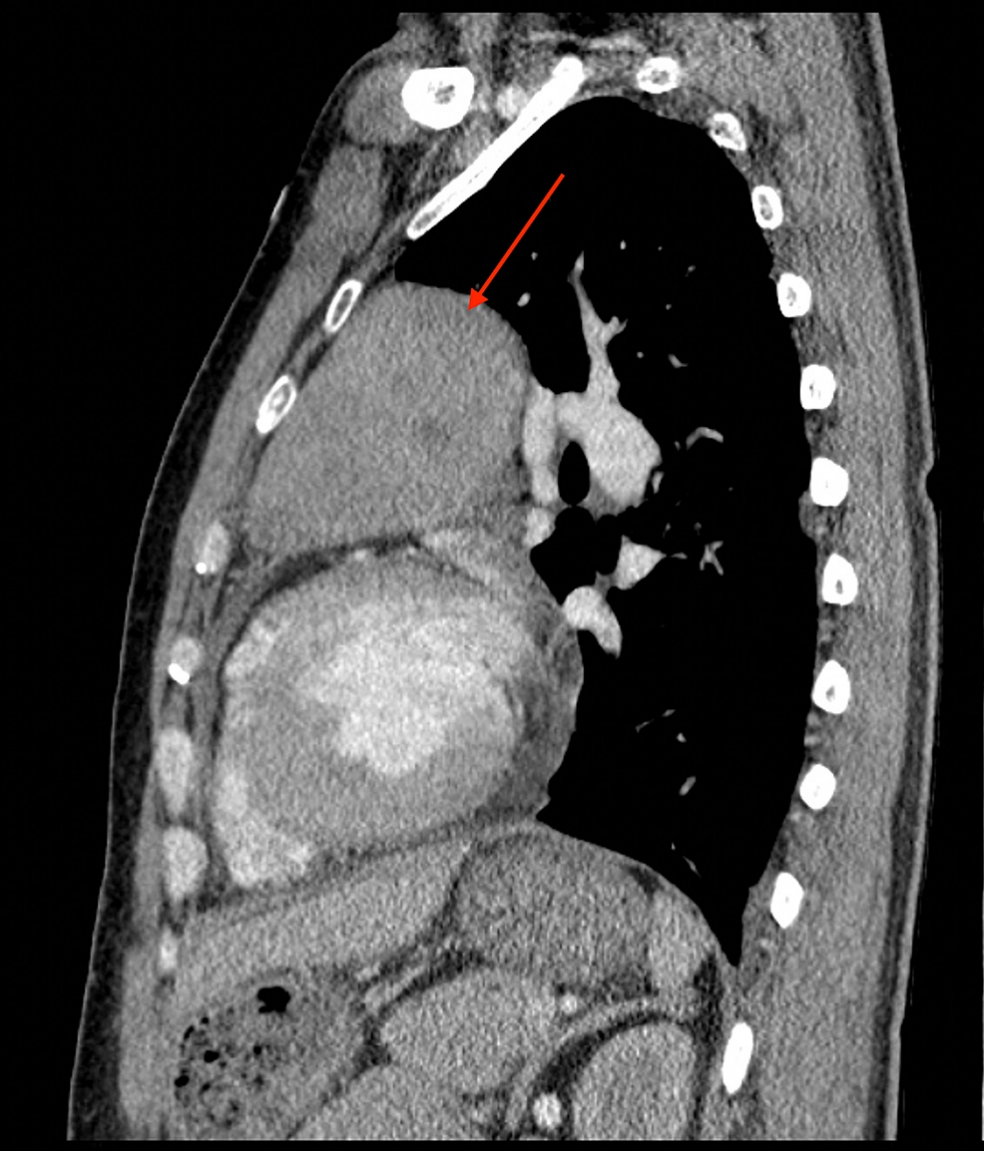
Chest-CT with contrast, sagittal view
